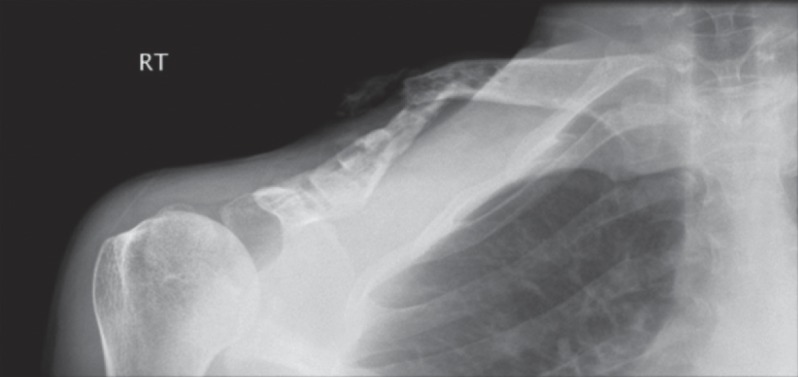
Radiograph showing patchy osteopenia, erosions, and no evidence of union.
